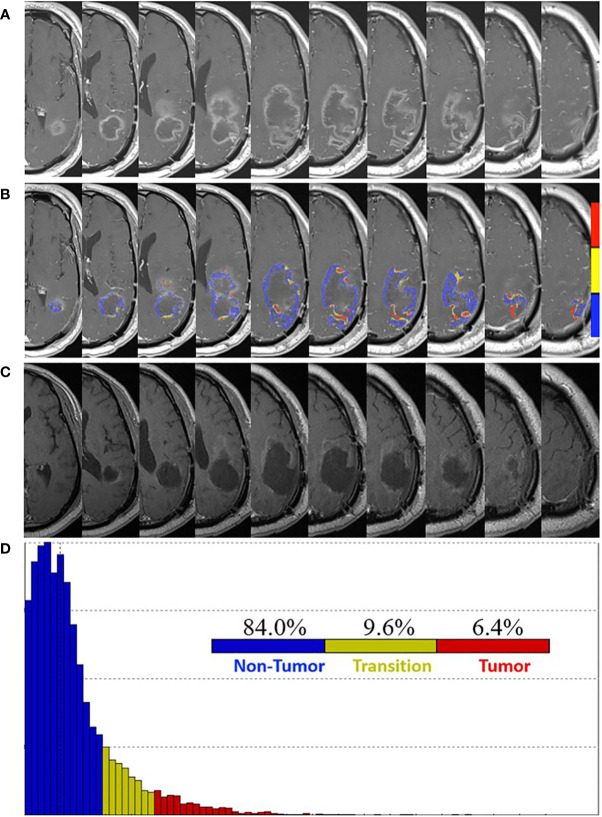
Pre- and post-surgery MRI. (A) Postcontrast T1-weighted MRI obtained five weeks post-pLDR and two weeks before redo-surgery. (B) Corresponding maps of FTB superimposed on the postcontrast T1-weighted MRI images. The FTB maps show areas of active tumor (red), treatment effect (blue), and transitional zone (yellow). (C) Post-surgery (four weeks) postcontrast T1-weighted MRI demonstrating extent of resection. (D) Distribution of T1-weighted enhancement volume based on vascularization as measured by FTB maps.
